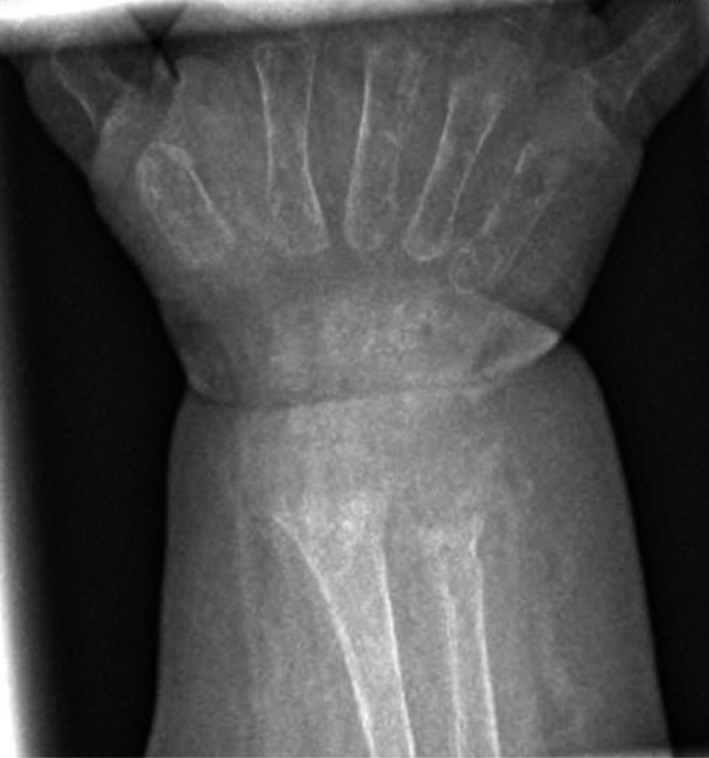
Radiographic image at admission of the wrist shows generalized osteopenia with cupping and fraying of metaphysis consistent with rachitic changes.
